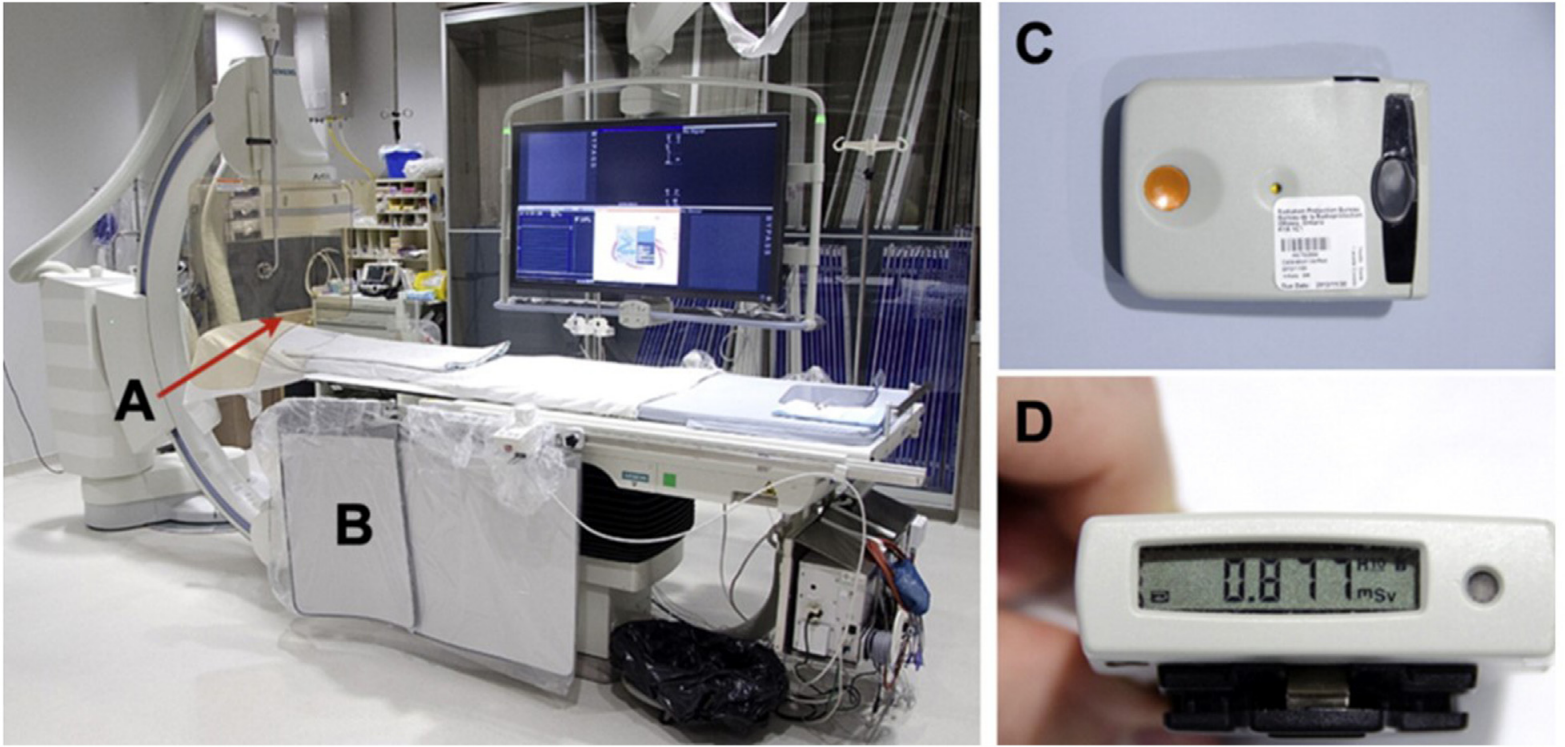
Novel RaySafe i2 system offers real-time x-ray radiation dose monitoring. (A) Ceiling-suspended acrylic shielding (1-mm lead equivalent), and (B) table-to-floor lead flap. (C) Electronic personal dosimeters worn by operators. An electronic personal dosimeter was worn on the left side of the thyroid lead shield and the recorded dose was absorbed by the operator during each case. (D) Electronic personal dosimeter screen display. From Abdelaal et al^22^ with permission from Elsevier.
